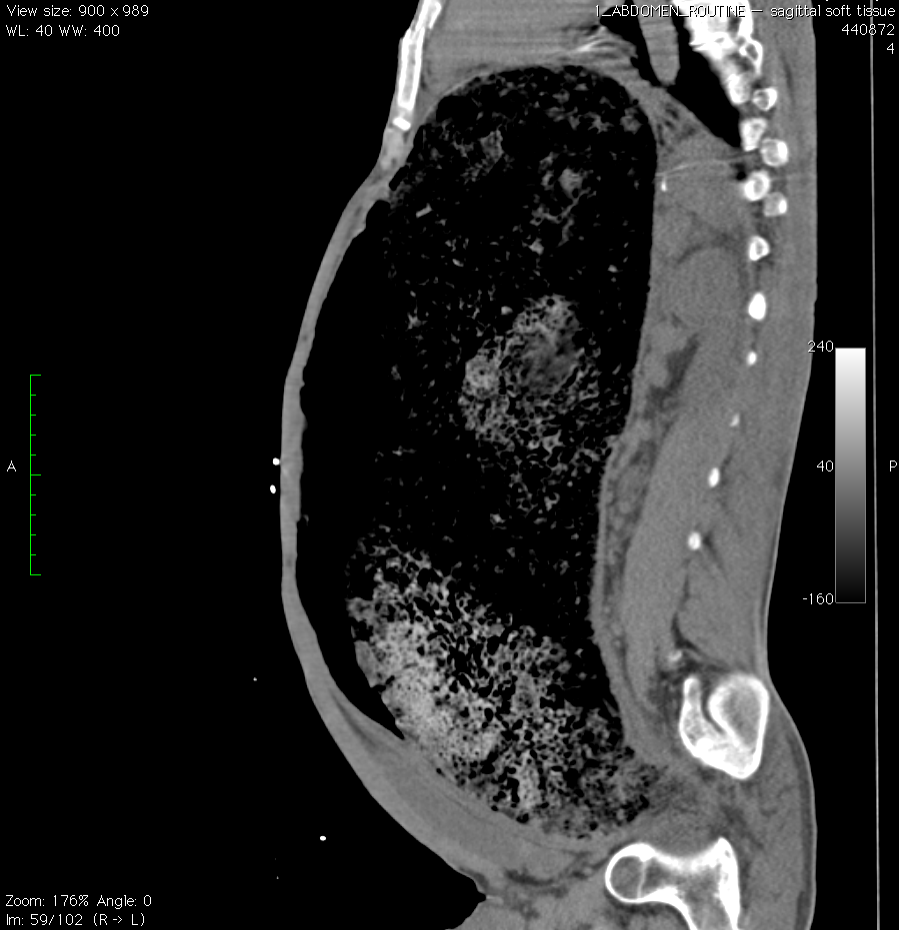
Sagittal CT image of the pseudo-obstruction.
